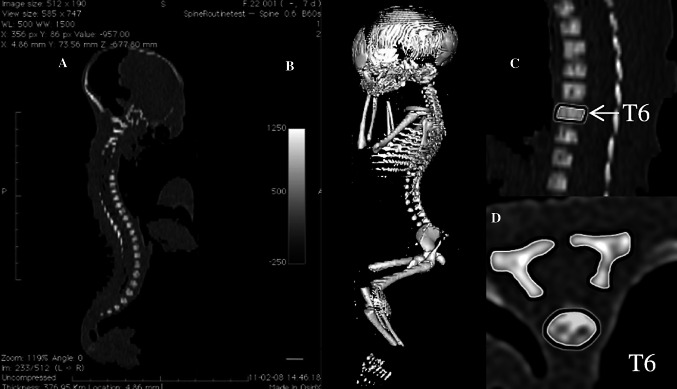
CT of a male fetus aged 23 weeks recorded in DICOM formats (*a*), and assessed by Osirix 3.9 (*b*) in both sagittal (*c*) and horizontal (*d*) planes (*red color* vertebral body, *green color* ossification center of vertebral body, *yellow color* ossification centers of neural processes) (color figure online)
